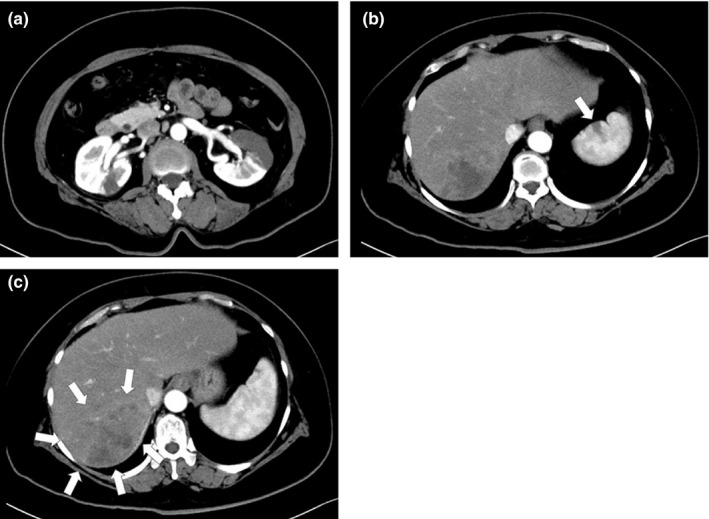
Image diagnosis of the case. (a) Contrast‐enhanced CT showed evidence of bilateral renal infarction. (b) Contrast‐enhanced CT showed splenic infarction (pointed by arrow head). (c) Contrast‐enhanced CT showed a tumor in the retrohepatic area(pointed by arrows heads)
